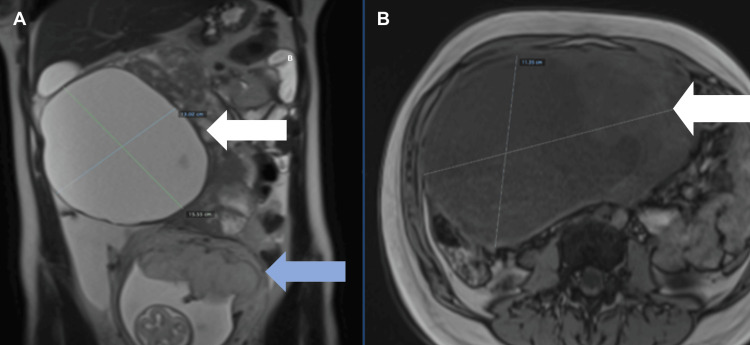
Abdominal-pelvic magnetic resonance imaging without contrast. (A) Coronal and (B) axial images. The white arrow points to the mass, and the blue arrow points to the fetus.
